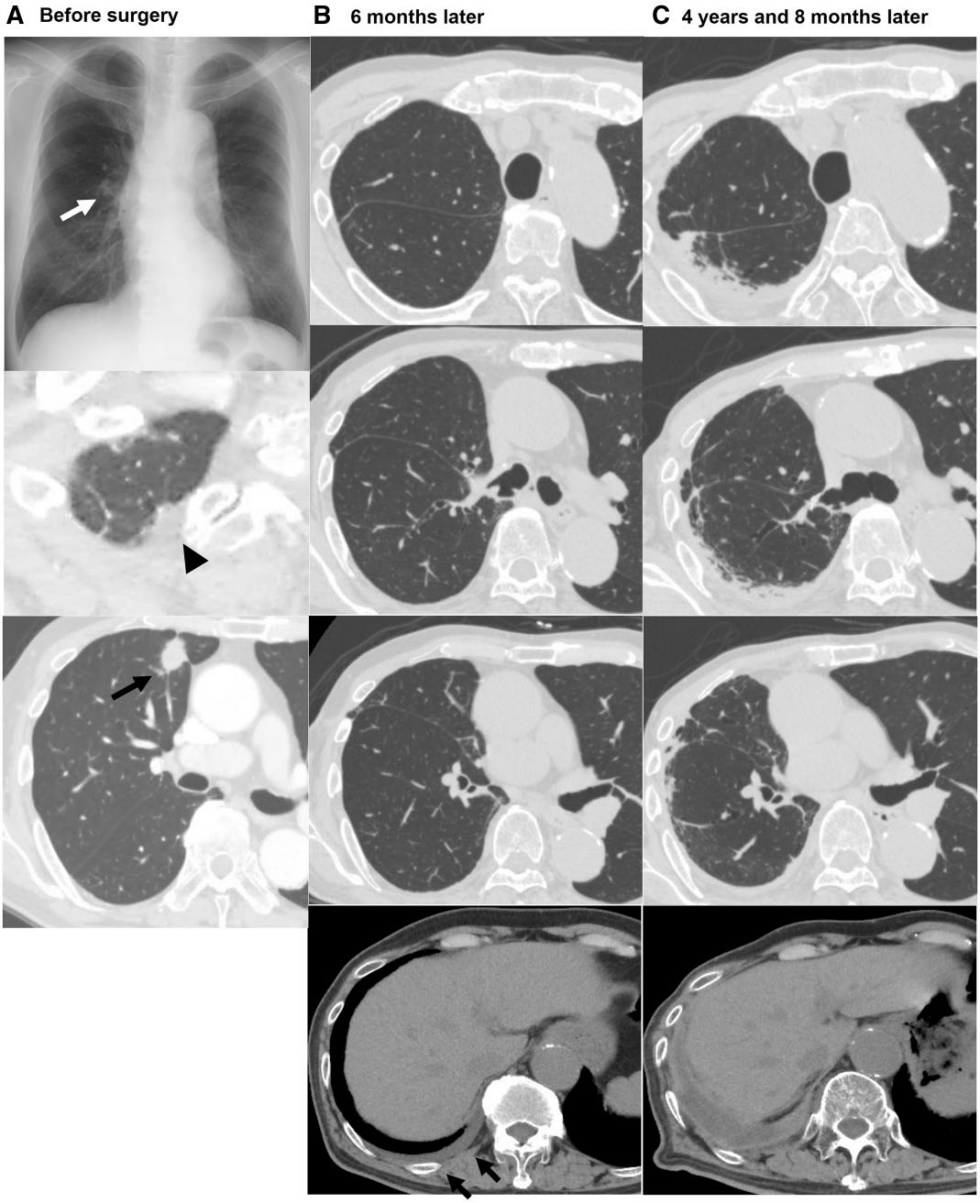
Radiological course of a 78-year-old male patient who underwent a right upper lobectomy for stage IB adenocarcinoma. (A) Preoperative radiological radiograph showed a small nodule (white arrow) in the right middle lung field, and chest computed tomography demonstrated a pulmonary apical cap (arrowhead) and a nodule (black arrow) in the right upper lobe. (B) Postoperative computed tomography at 6 months after surgery showed no abnormal shadows except for a small amount of pleural effusion (arrow) on the operated side. (C) At 4 years and 8 months after surgery, pleural thickening and subpleural fibrosis lesion emerged in the right upper lung field with unilateral thoracic deformity and thickened pleural effusion.
